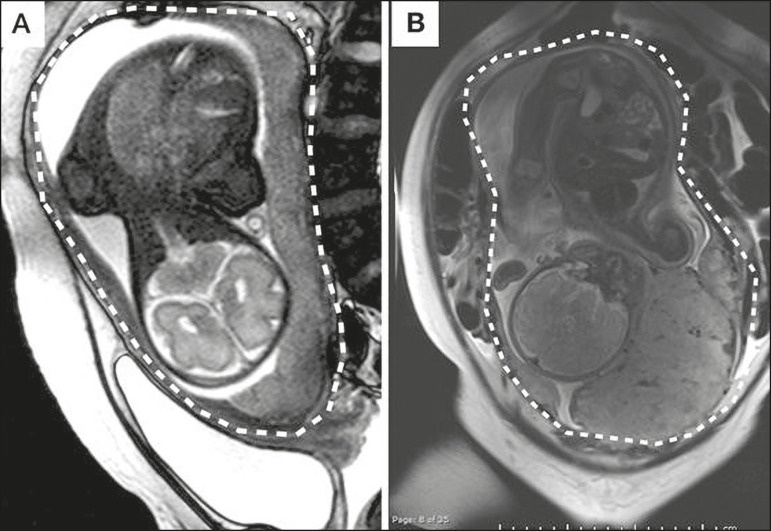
A: Sagittal T2-weighted MRI sequence showing a gravid uterus of normal appearance (inverted-pear shape) with no features of placenta accreta. B: Coronal T2-weighted MRI sequence showing a uterus with an hourglass shape, suggesting a diagnosis of placental adhesion disorder.
